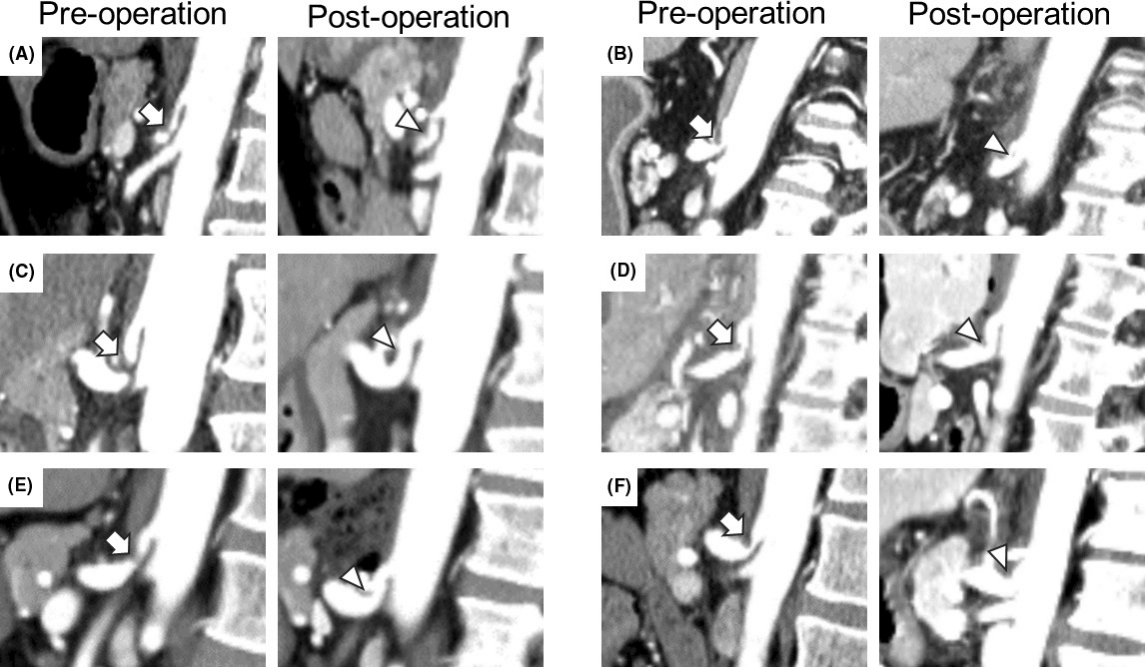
Postoperative assessment with contrast‐enhanced CT. Left panels, preoperative contrast‐enhanced CT showing stenosis or obstruction of the CA (arrow); Right panels, postoperative contrast‐enhanced CT showing dilation of the CA (arrowhead). (A) Case 1; (B) Case 2; (C) Case 3; (D) Case 4; (E) Case 5; (F) Case 6. CA, celiac artery; CT, computed tomography.
